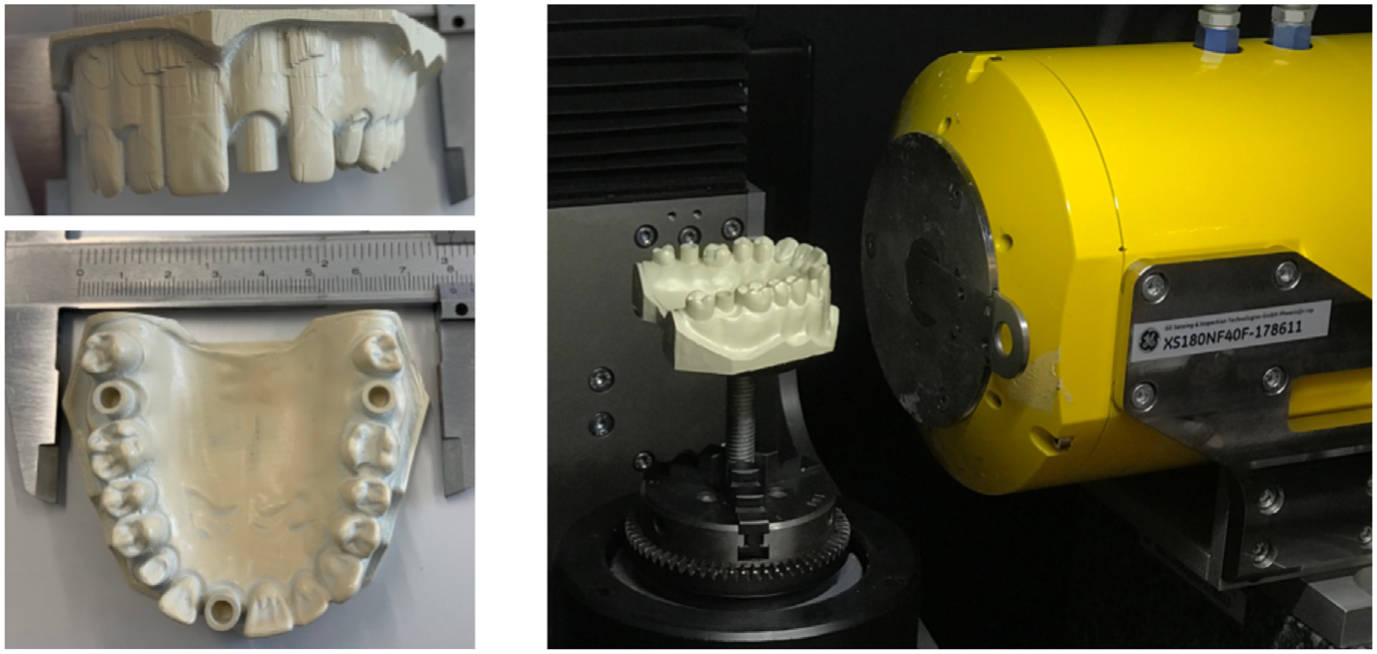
The PEEK model’s size corresponds to that found in the human body, as displayed by the photographs on the left. Three well-defined hollow cylinders were incorporated to determine the precision of the IOSs. The other photograph shows the placement of the model on the rotation stage in the CT-system nanotom^®^ m (phoenix|x-ray, GE Sensing & Inspection Technologies GmbH, Wunstorf, Germany).
